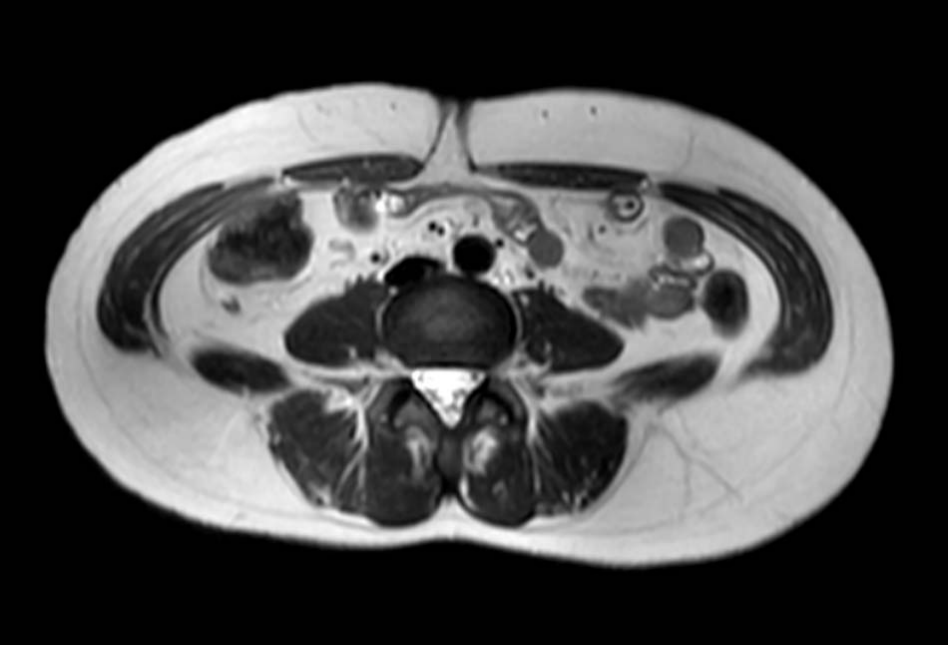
MRI axial view of the port site hernia.
